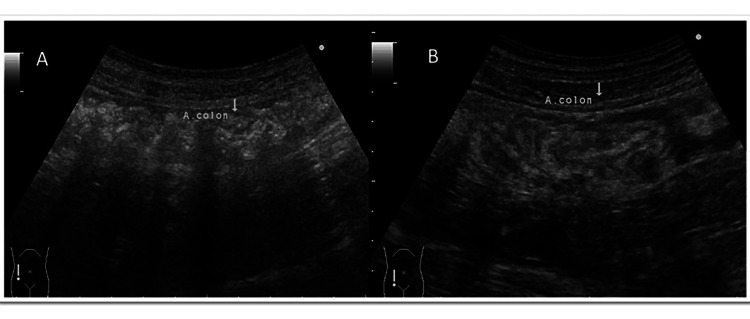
Abdominal ultrasound images of this case The abdominal ultrasound (US) images were taken in the right lower abdomen of the patient. (A) Intestinal edema was noted from the terminal ileum to the hepatic curvature of the transverse colon. Ascites was present around the ileocecal region. (B) Pseudo-kidney sign was observed.
